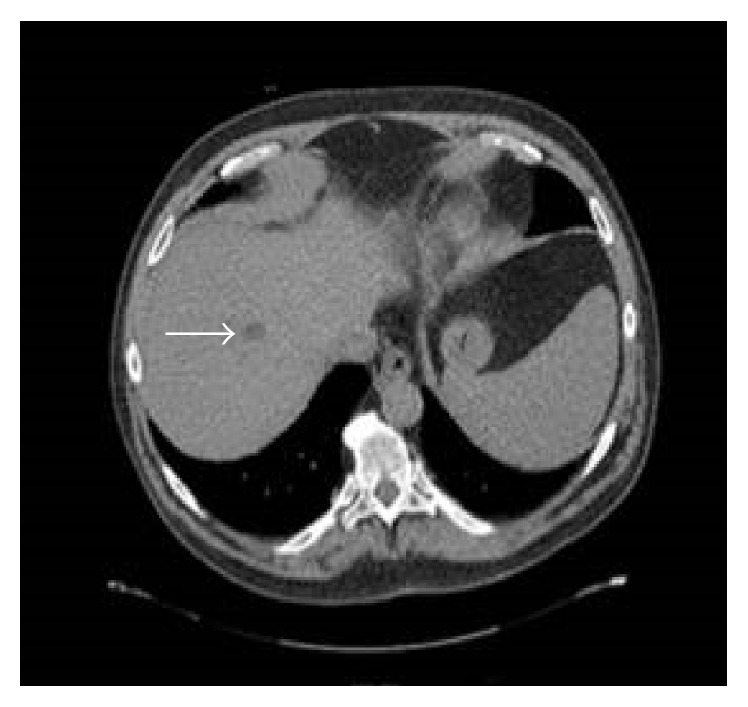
Multidetector CT of the abdomen on the day of admission showed a new ill-defined hypodense lesion in the right hepatic lobe.
